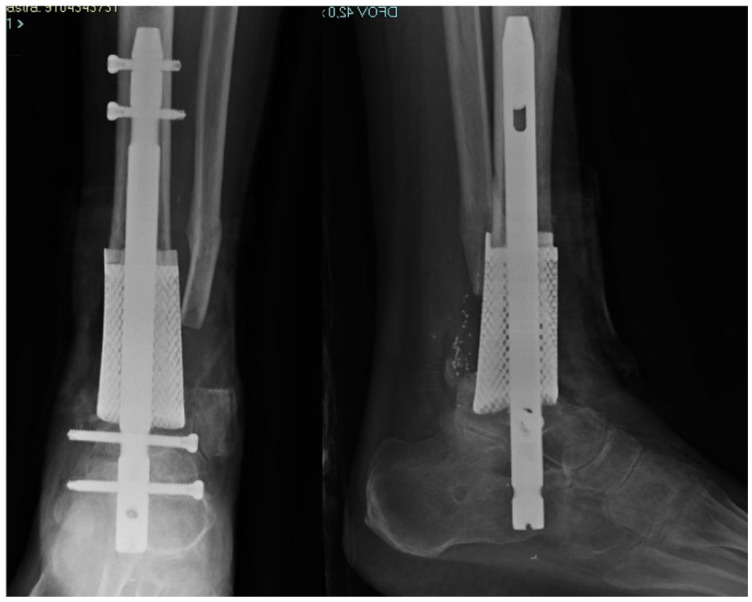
Post-operative X-ray of the final custom-made construct.
